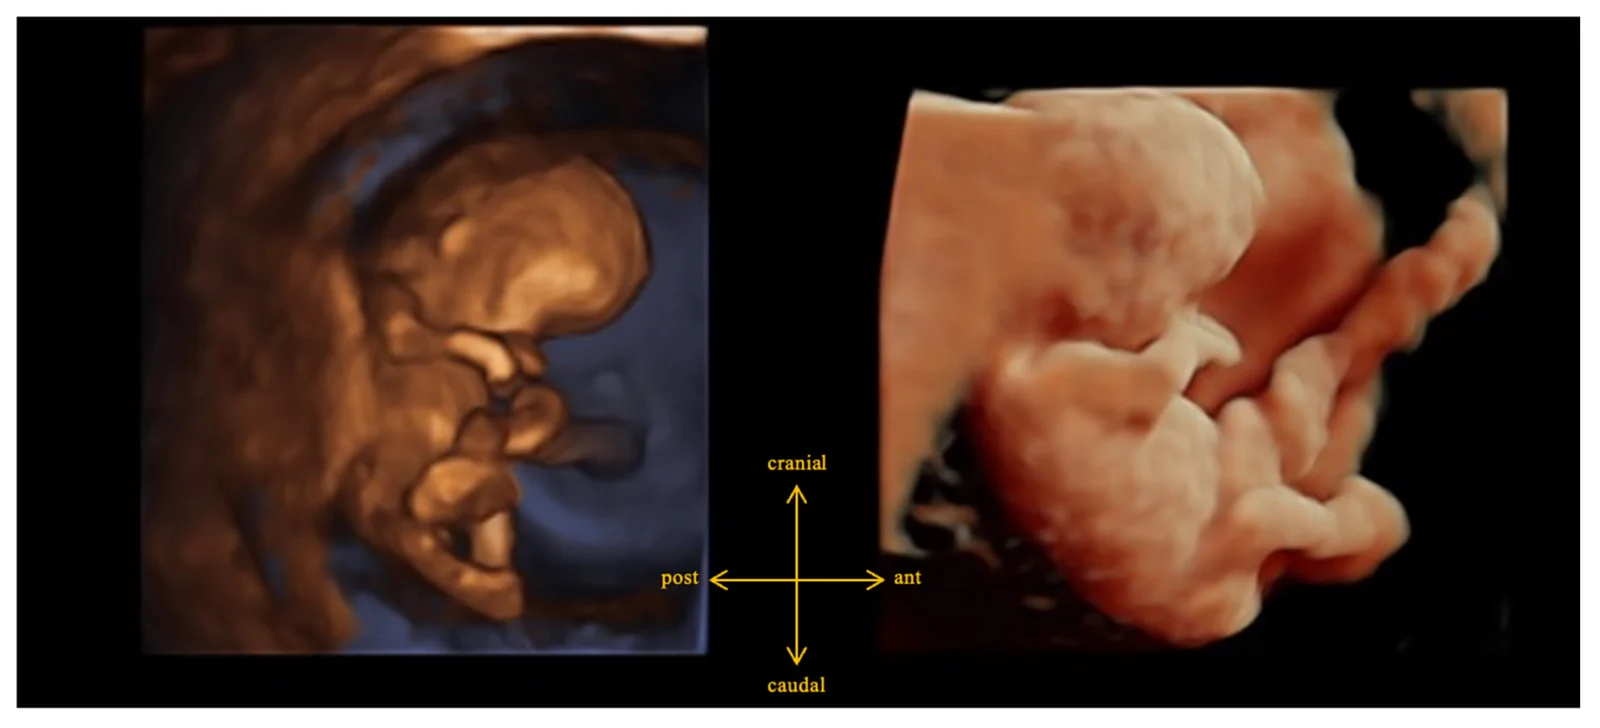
Ultrasonographic aspects of 10 weeks of gestation individuals—development of the lower limbs along with the position of the umbilical cord hinders the approach of the genital tubercle during ultrasound assessment.
